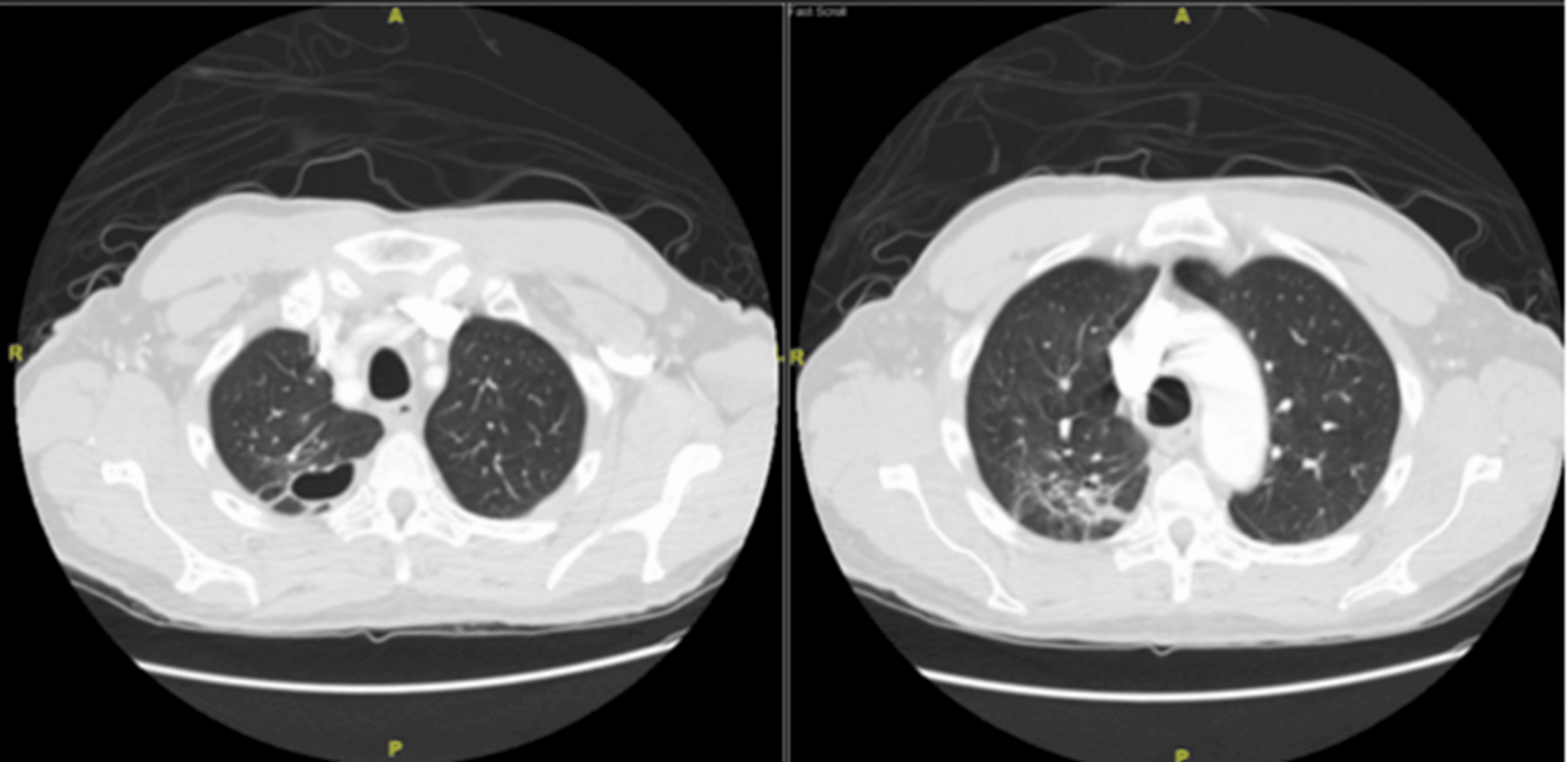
CT chest after completion of treatment Cavitary lesion on the right upper lobe (left image) and reduced consolidation in the right lower lobe (right image)
